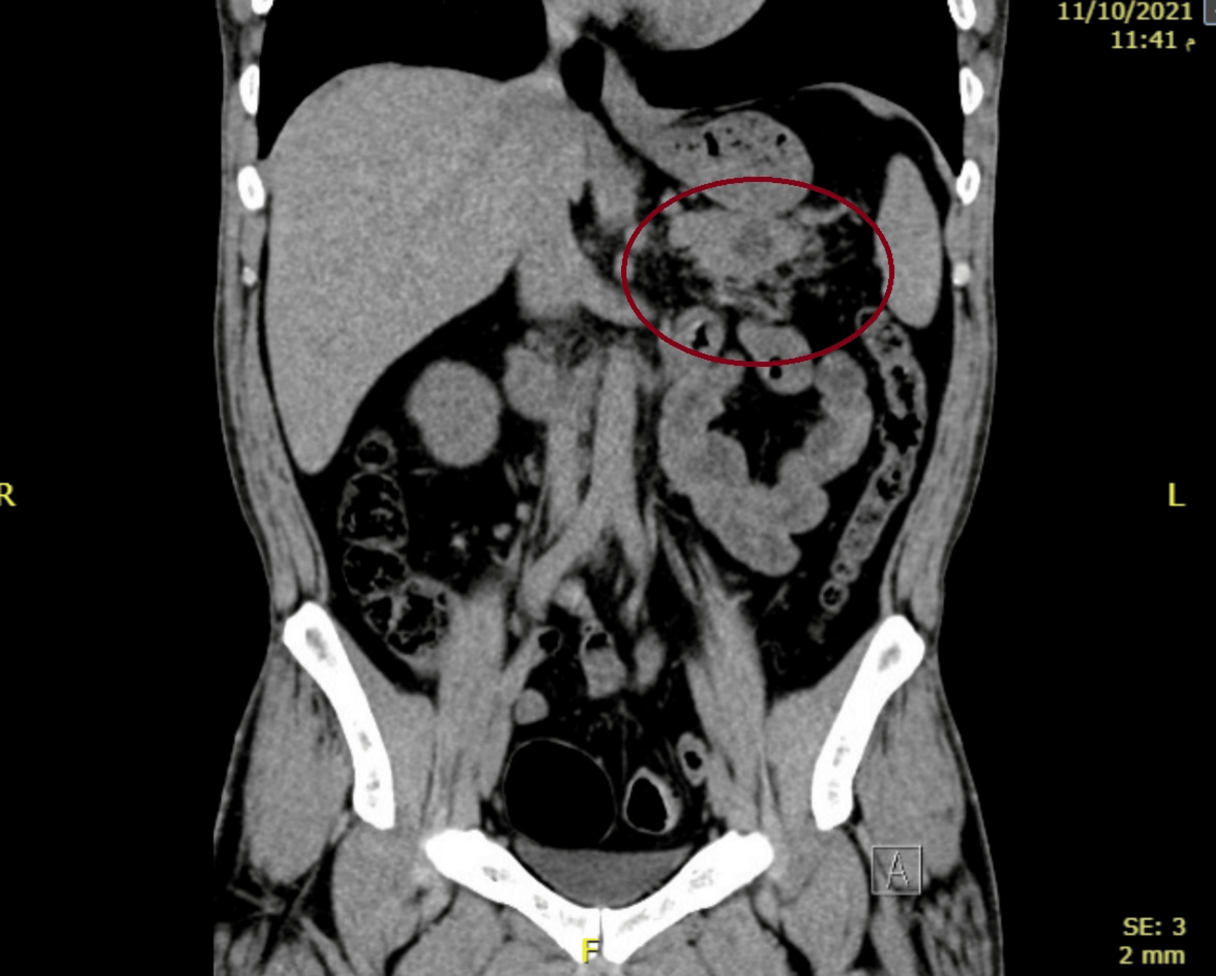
An unenhanced CT scan of the abdomen and pelvis revealing a hypodense lesion seen at the pancreatic tail associated with mild peripancreatic fatty stranding.
